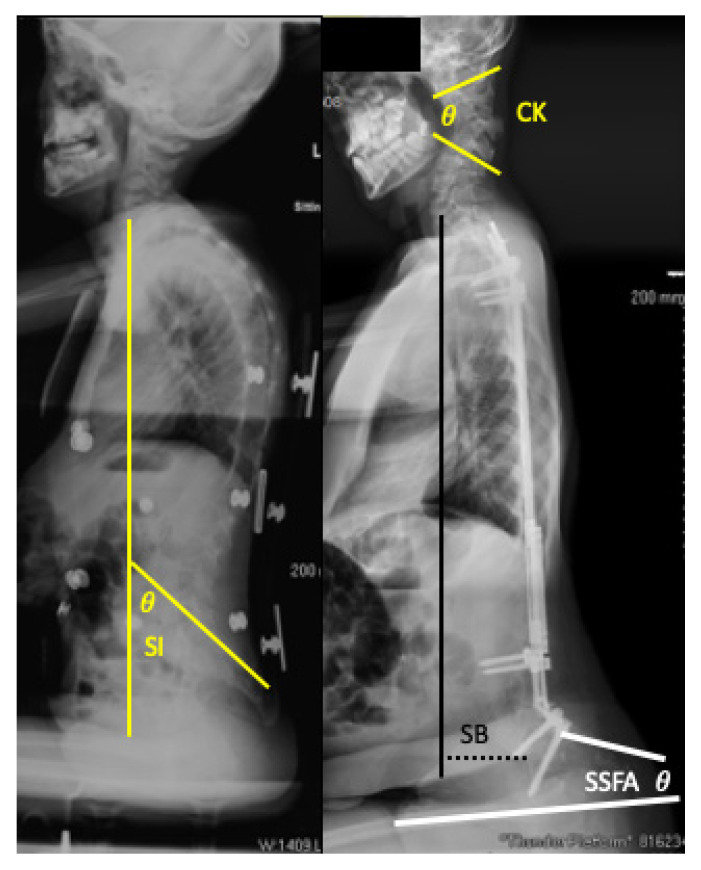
Examples of radiographic sagittal parameters used to objectively characterize deformities. These include Sacral Incidence (SI, Yellow); Cervical Kyphosis (CK, Yellow); Sagittal Balance (SB, Black); Seated Sacral Femoral Angle (SSFA, White).
